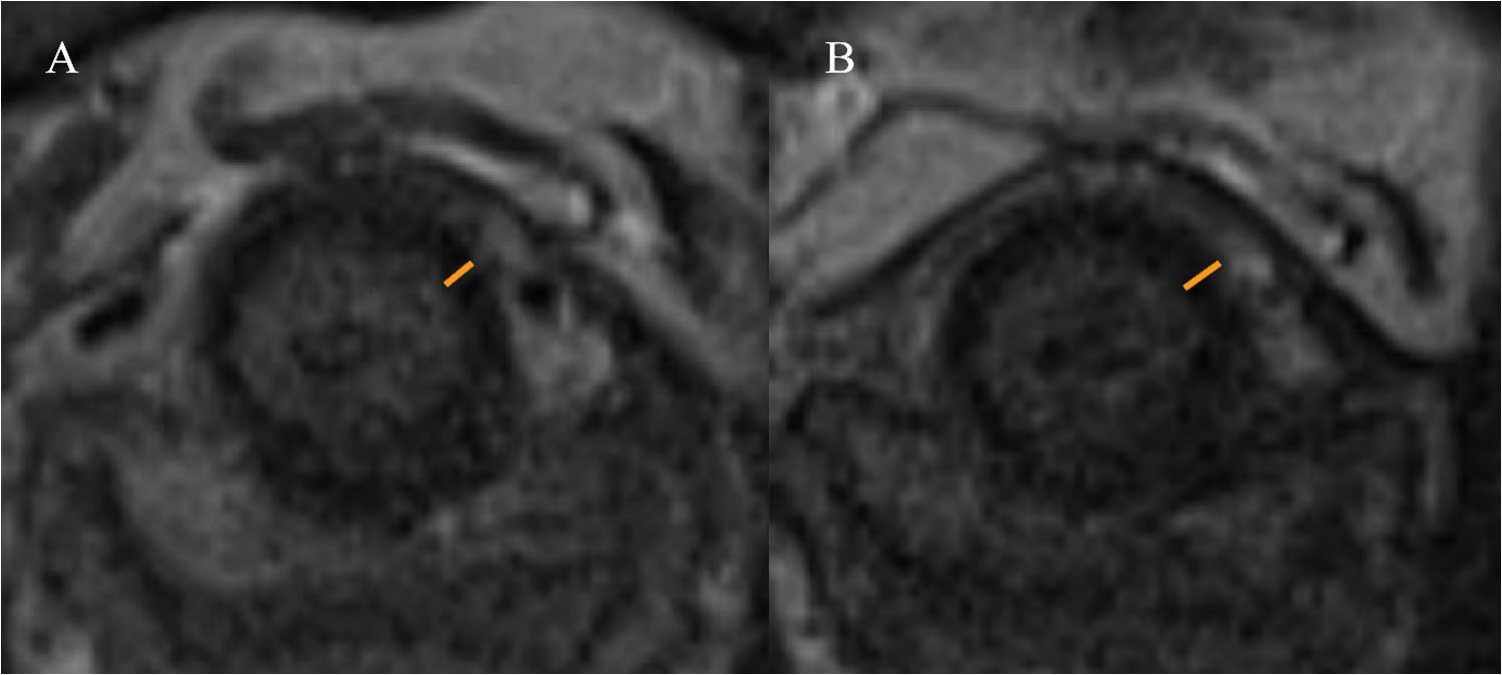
MRI of the pelvis with a T2-weighted sequence in axial and sagittal orientation for the anatomical analysis of the external urinary sphincter (*EUS*) diameter, no presence of necrosis or tumour at A baseline and B follow-up
